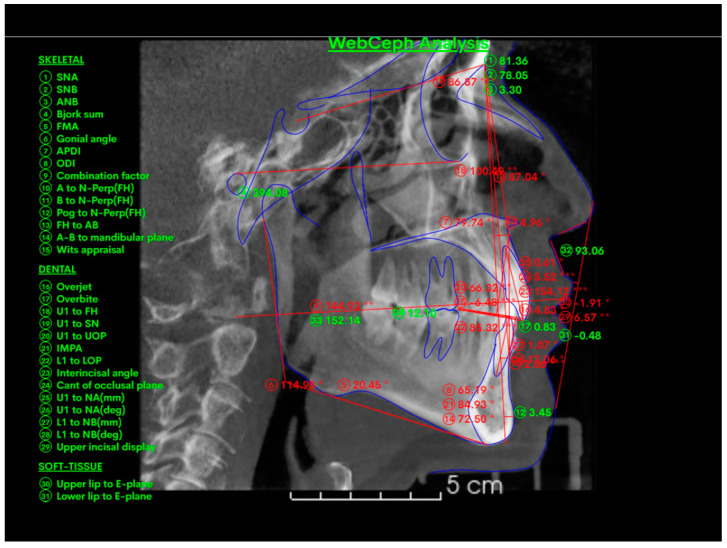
Cephalometric measurements in 2D. Footnote: cephalometric measurements in 2D were performed on a lateral radiograph derived from cone beam computed tomography (CBCT) from an orthogonal view, free of distortion. Using the Webceph program, values were obtained for the interincisal angle, skeletal class, overjet, and overbite. The measurements marked with * indicate ±1 clinical deviation from the norm, while  indicates ±2 clinical deviations from the norm.
